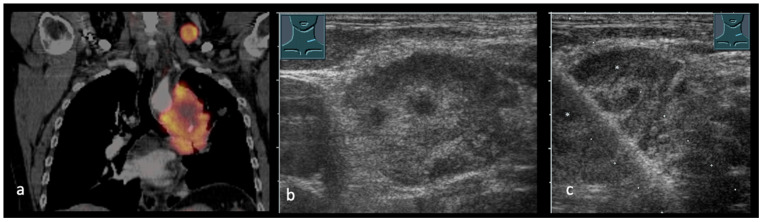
(a–c): Central lung cancer and suspected supraclavicular lymph node metastasis in PET-CT-scan (a). B-US presents a supraclavicular lymph node with complex echogenicity with tracer accumulation in PET-CT (b). Ultrasound guided biopsy of the suspected lymph node (c).
